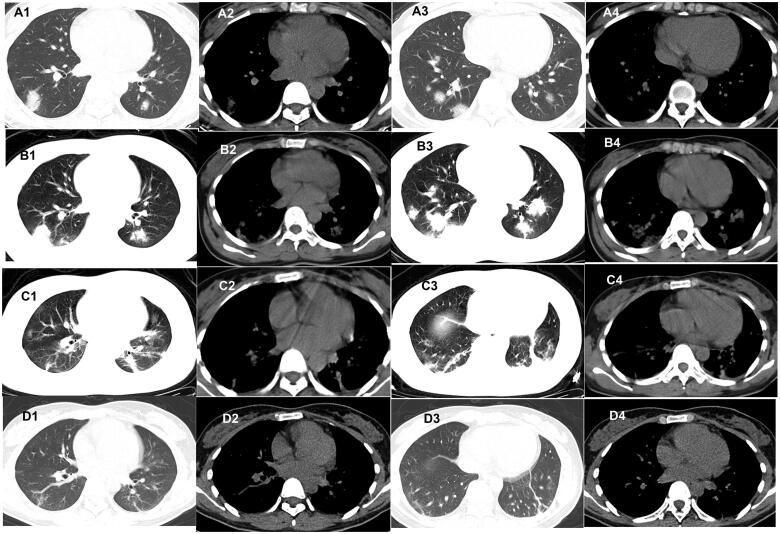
Evolution of the nodules on chest CT of patients with COVID-19. A 27-year-old woman who had close contact with individuals in Wuhan presented with fever and fatigue for 3 days. (A1–A4) The first non-contrast-enhanced chest CT reveals multiple nodules in the left and right lower lobes (initial chest CT). (B1–B4) Follow-up chest CT 4 days after the first shows that both the scope and density of the lesions increase (stage I*); (C1–C4) Follow-up chest CT 8 days after the first shows that both the scope and density of the lesions decrease (stage II*). (D1–D4) Follow-up chest CT 12 days after the first shows that the lesions are almost absorbed completely (stage III*). *The stage does not represent the course of COVID-19 but the time interval between the two adjacent CT scans.
